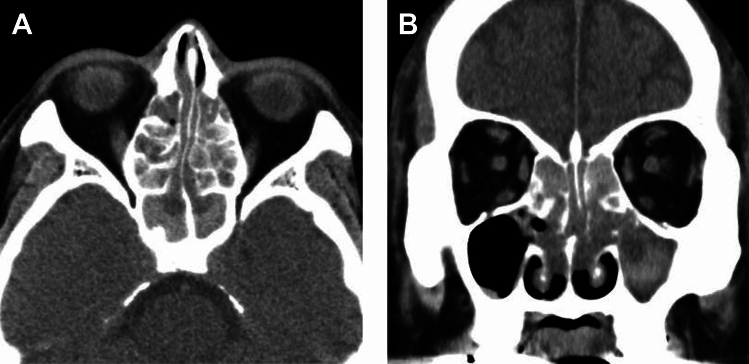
Sinonasal involvement in a 62-year-old man with eosinophilic granulomatosis with polyangiitis. (A) Axial and (B) coronal CT show diffuse sinus opacity with hyperdense areas, and nasal polyps
